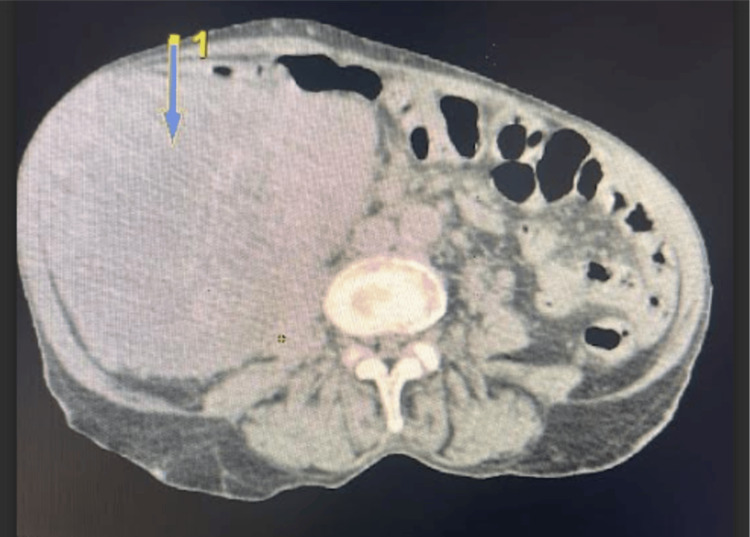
CT Scan Showing Right-Sided Renal Cell Carcinoma Showing Mass Effect on the Liver
